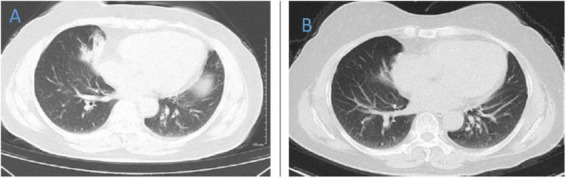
Chest CT of patient 8. (A) Before treatment on 2022.1.10. (B) The follow-up imaging 1 week after treatment showed improvement compared to the pre-treatment scans.
